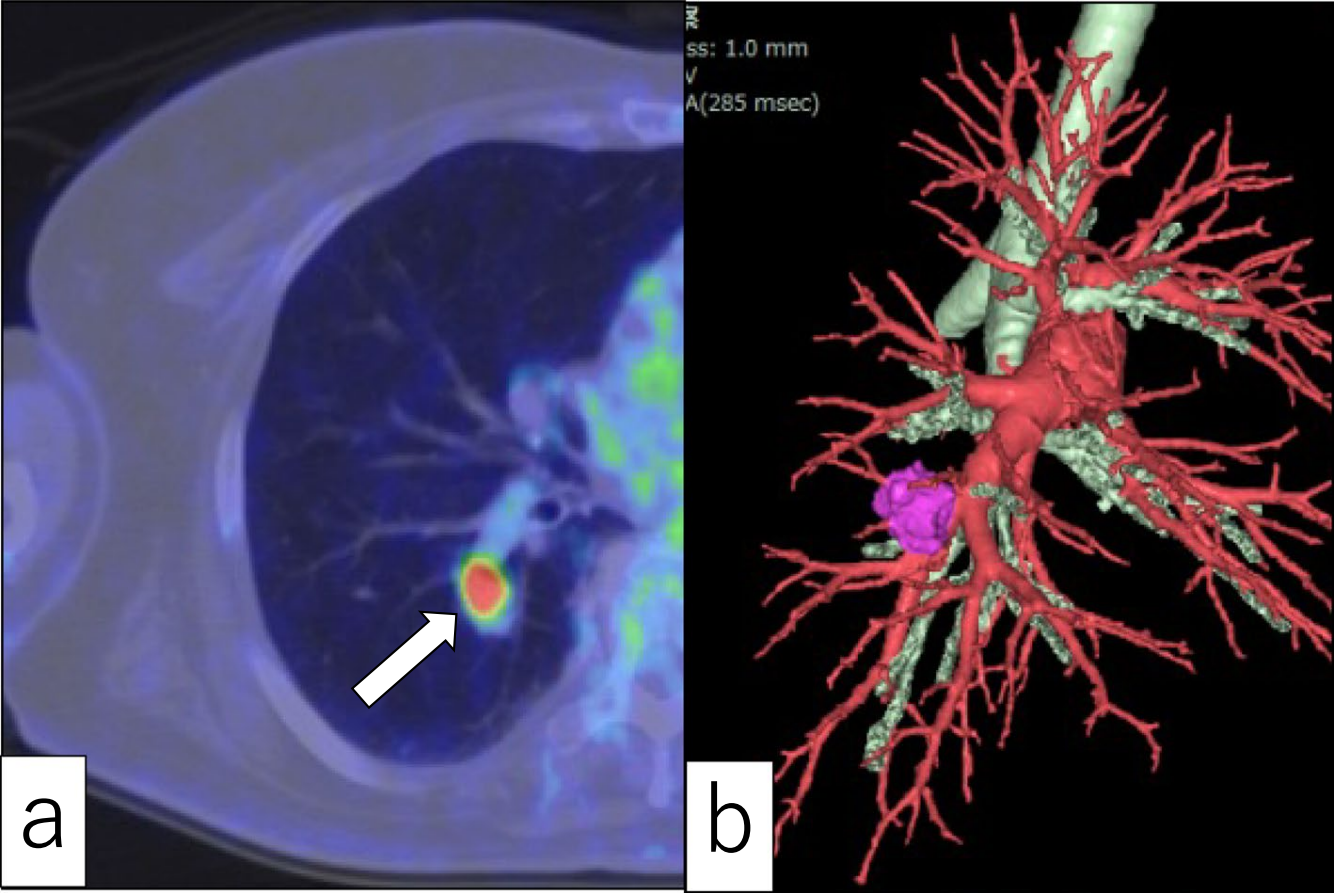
a Axial FDG-PET-CT fusion showed a nodule (arrow) with high FDG uptake (SUV_max_ 13.01) in the right lower lobe of the lung. b 3D-CT of the right pulmonary artery and bronchus confirmed that the tumor was located in the pulmonary center. Tumor, pink; bronchus, white; arteries, red
